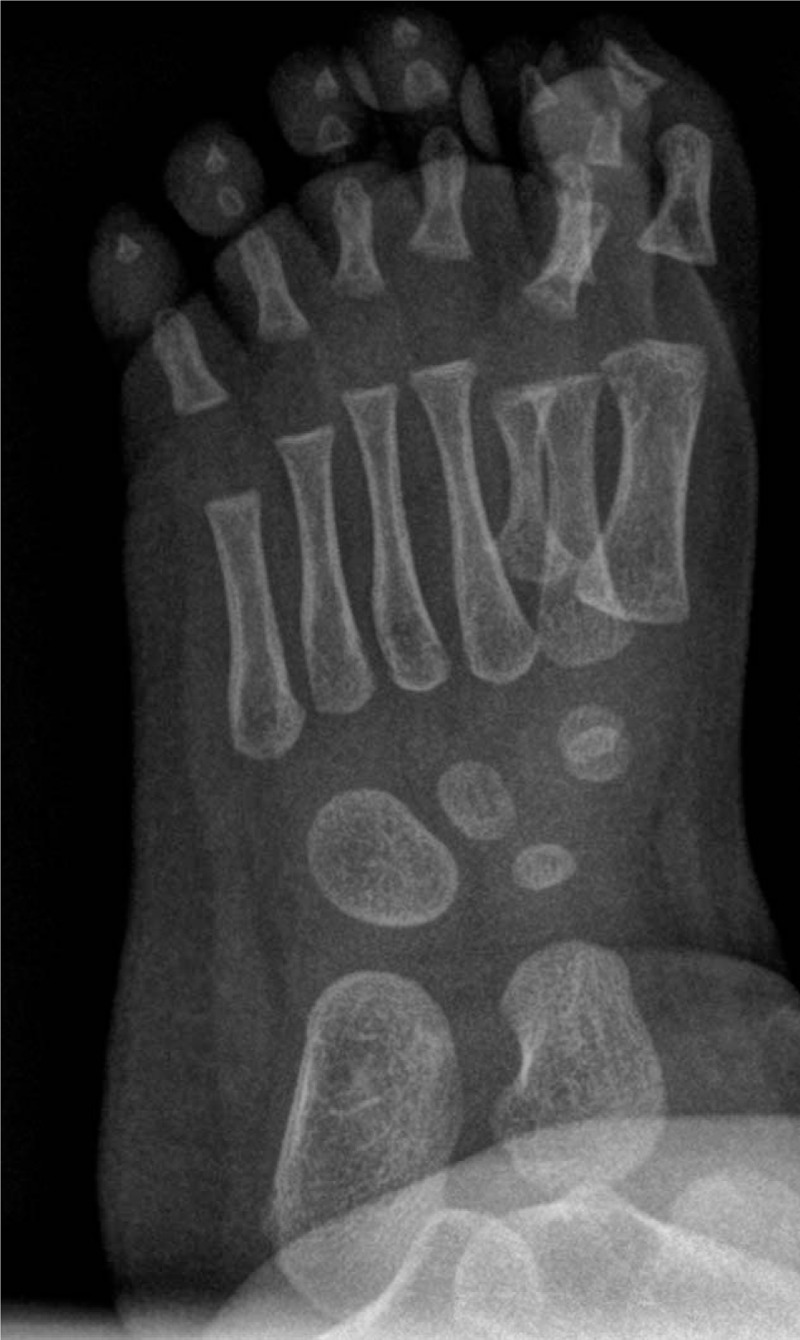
Foot radiographs revealed the presence of 7 complete rays (oblique plane).
